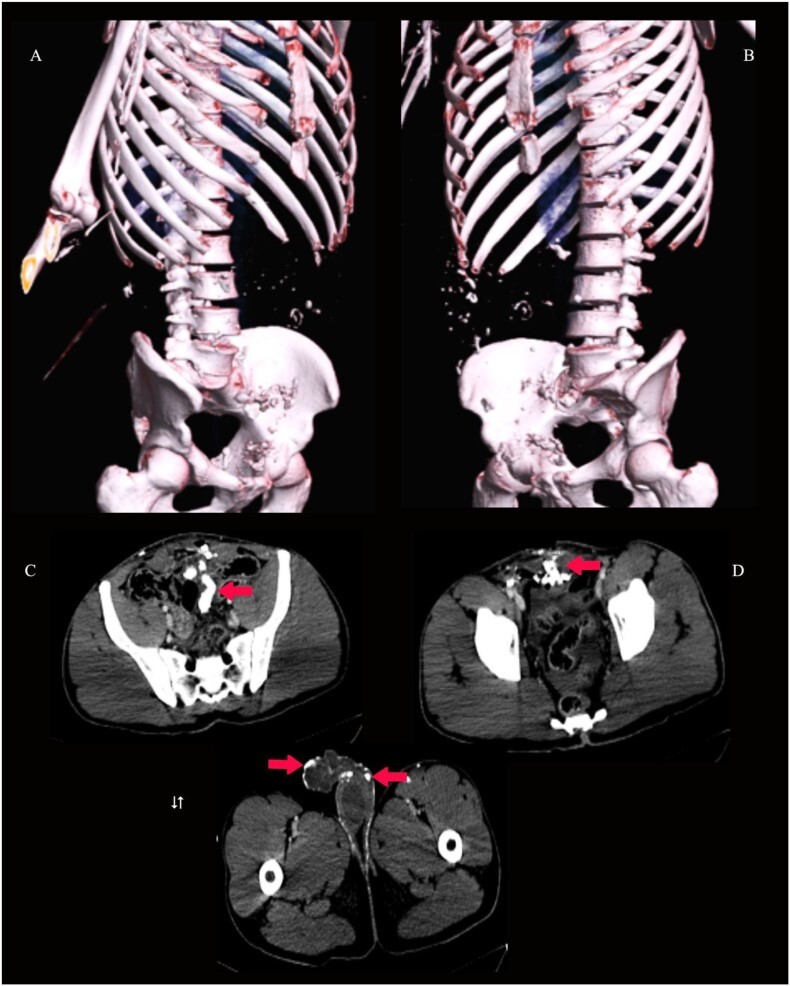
3D virtual reconstruction images (A and B) and axial CT pelvis (C, D and E) show innumerable radio-opaque foreign bodies (shrapnel) in the peritoneal cavity, anterior rectus abdominis muscle and the subcutaneous tissue of the penis, scrotum and left thigh, as shown by arrows.
